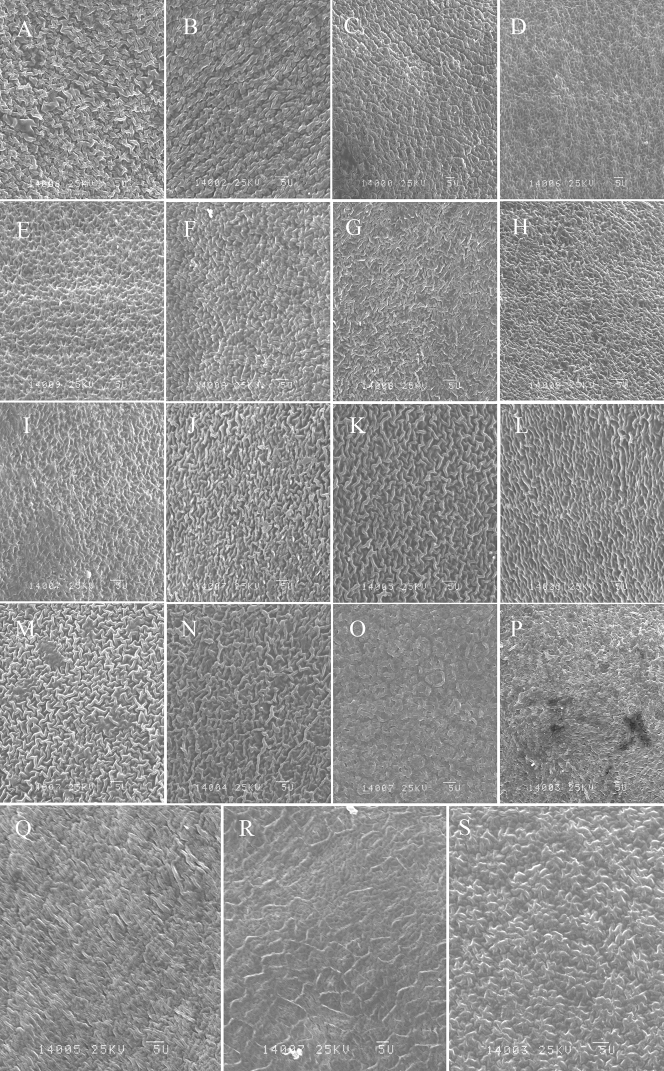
Seed surface sculpturing of the studied species A*O.coerulea* (TT) B*O.coerulea* (EDG) C*O.holanshanensis*D*O.xinglongshanica* (MX) E*O.xinglongshanica* (XL) F*O.glabra*G*O.kansuensis* (AZ) H*O.kansuensis* (CT) I*O.taochensis*J*O.ochrocephala* (NH) K*O.ochrocephala* (XL) L*O.ochrocephala* (MX) M*O.ochrocephala* (JQ) N*O.ochrocephala* (HG) O*O.qinghaiensis*P*O.latibracteata*Q*O.qilianshanica*R*O.aciphylla*S*O.squammulosa*. Scale bar: 5 μm.
